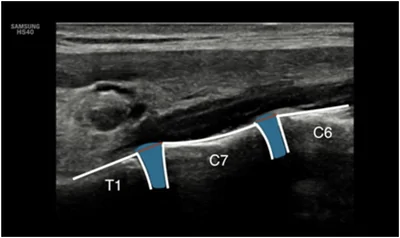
Longitudinal plane ultrasonic image of C6–C7 and C7–T1 cervical intervertebral disc (IVD) spaces with markers placed at the most superior anterior point of the inferior vertebrae and the most inferior anterior point of the superior vertebrae to facilitate anterior IVD height assessment.
